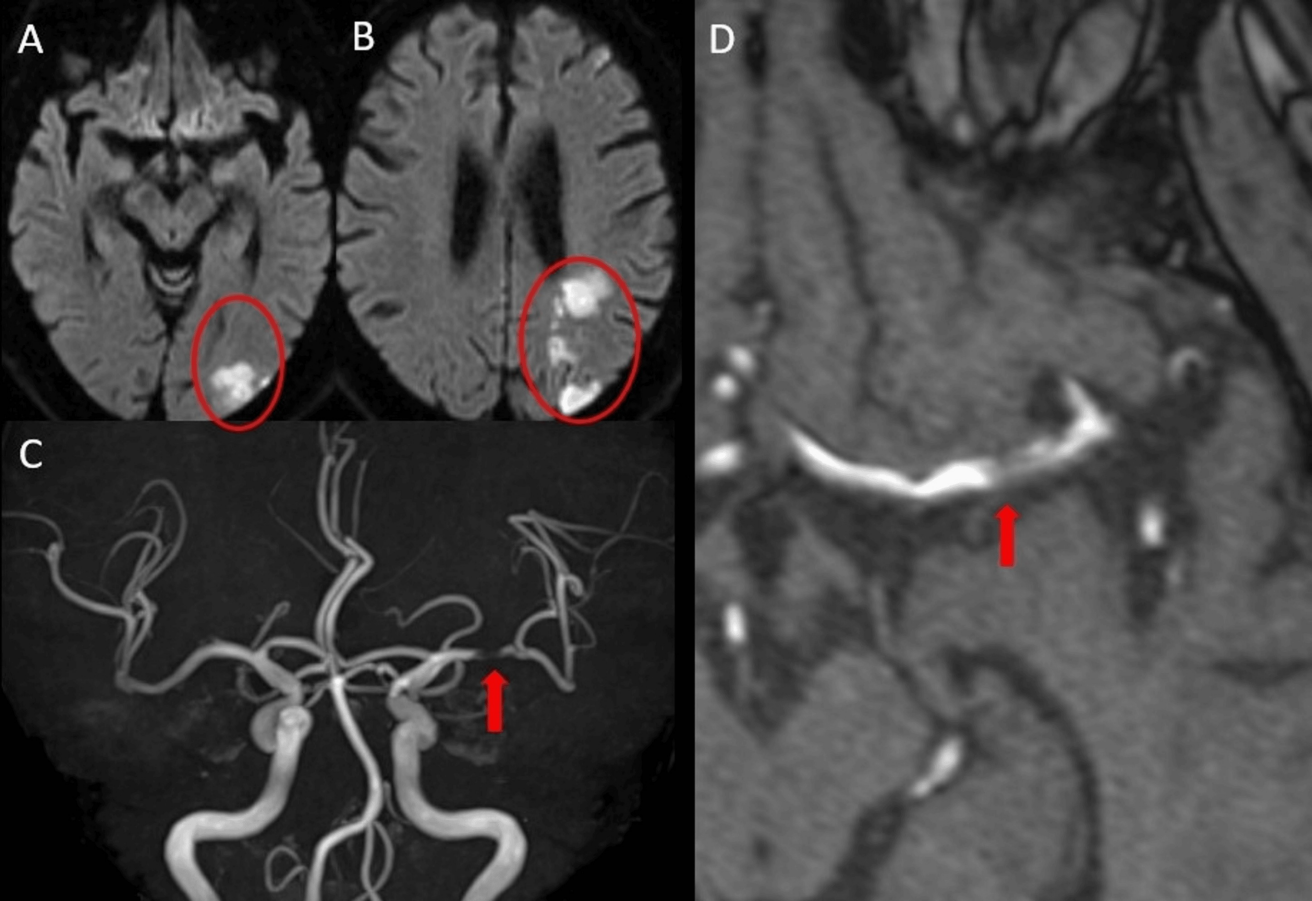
Case 2: Imaging progression on hospital day 5 (A, B) Follow-up DWI showing exacerbation and expansion of the known infarcts following clinical deterioration (red ovals). (C, D) MRA demonstrating progression of the dissection and stenosis compared to admission imaging (arrow). DWI, diffusion-weighted imaging; MRA, magnetic resonance angiography
